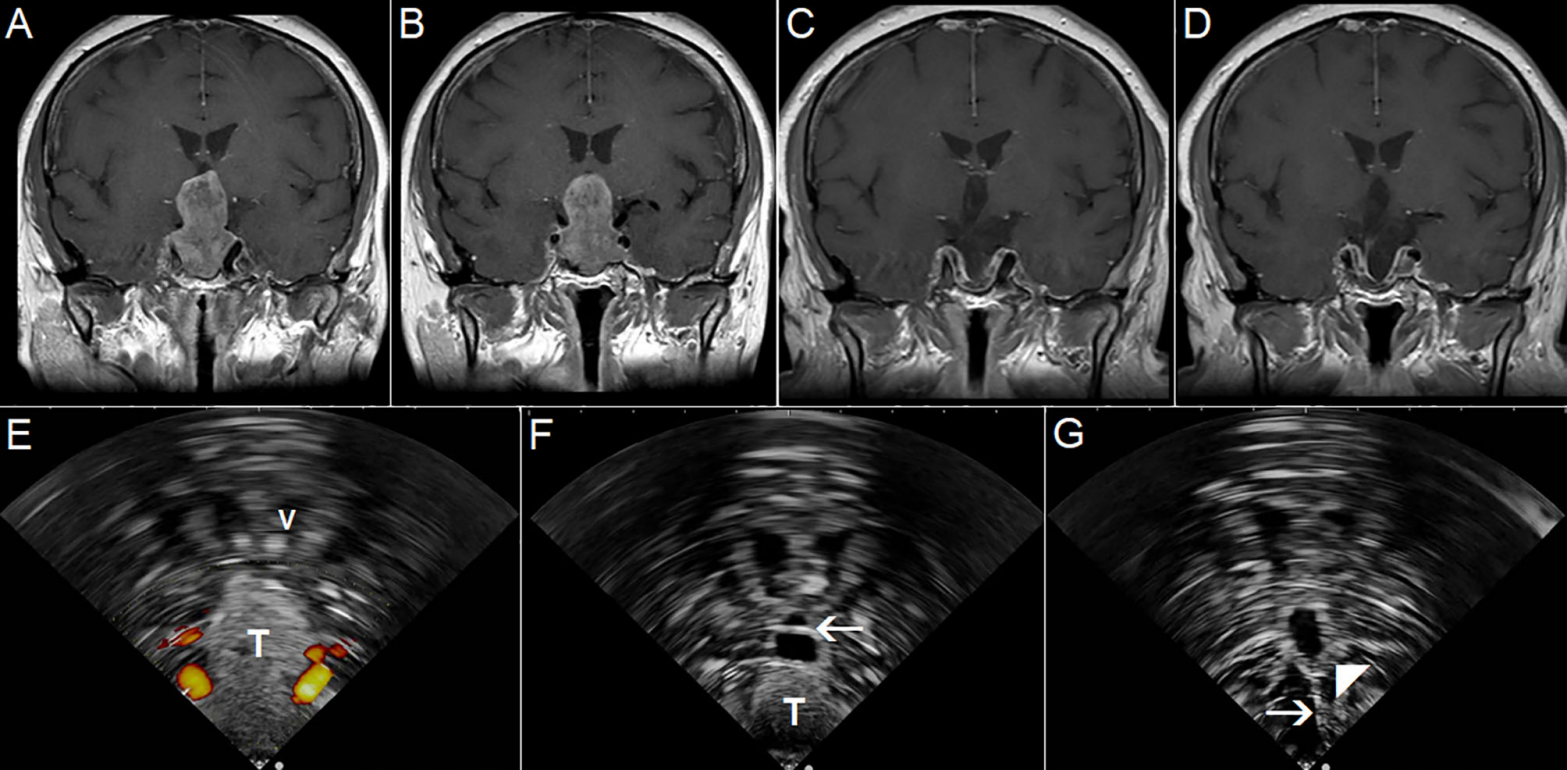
Pituitary macroadenoma invading left cavernous sinus (A, B) Preoperative coronal contrast-enhanced T1 MRI sequence (C, D) Postoperative coronal contrast-enhanced T1 MRI sequence, small tumor residuum was intentionally left in the left cavernous sinus (E) Pre-resectional 2D IOUS image using flexible mini-probe, note distinct depiction of the tumor tissue as well as visualization of upper segments of carotid arteries by power-Doppler mode (F) Intraoperative 2D IOUS image after partial tumor resection. Note partial decompression of the third ventricle with depicted interthalamic adhesion (arrow) (G) 2D IOUS image after tumor resection, note pituitary stalk is distinctly visible (arrow) as well as the floor of the 3rd ventricle (arrowhead). 2D, two-dimensional; MRI, magnetic resonance imaging; IOUS, intraoperative ultrasound; V, lateral ventricle; T, tumor.
